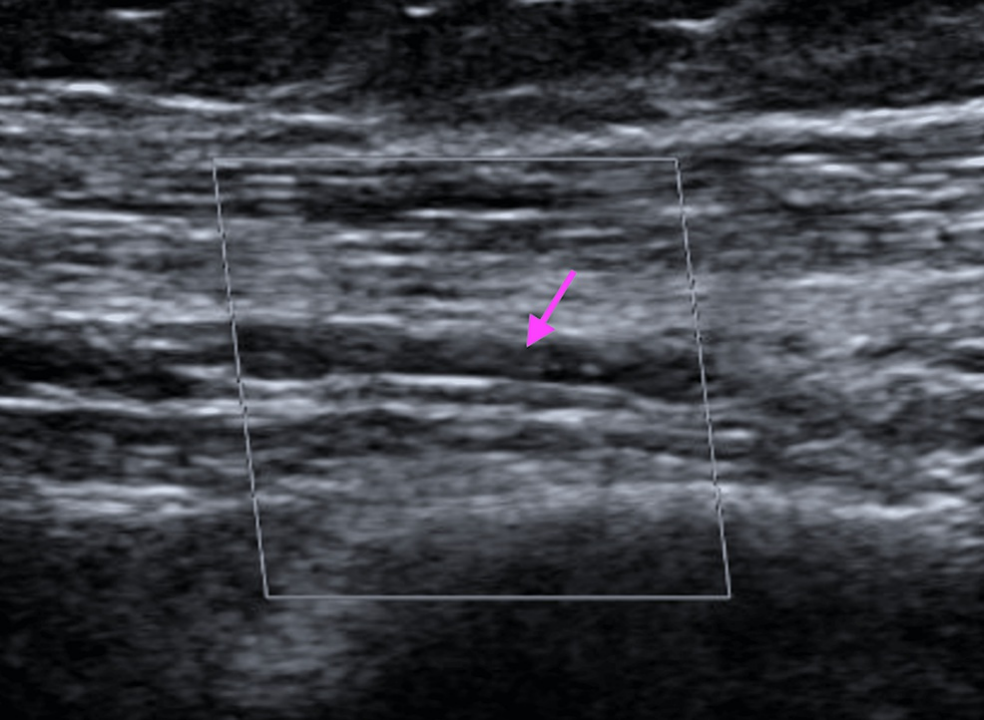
Nonocclusive DVT involving the right mid-brachial vein on ultrasound in Case 3 DVT: deep vein thrombosis
